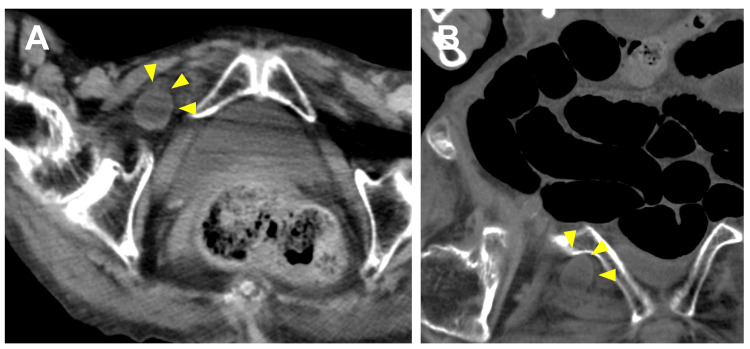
Case B: Preoperative CT. (A) Axial view showing right obturator hernia with incarcerated terminal ileum (arrowhead). (B) Coronal view.
